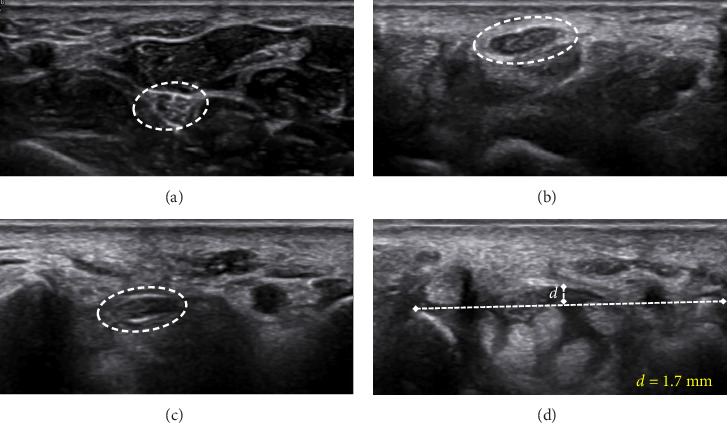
Transverse ultrasound image of the right median nerve in a female patient with mild CTS. (a) CSA at the pronator quadratus muscle (CSAp) is 0.06 cm^2^. (b) At the carpal tunnel inlet, CSA increased to 0.11 cm^2^. (c) The CSA at the carpal tunnel outlet is 0.09 cm^2^. (d) Bowing of the flexor retinaculum at the inlet is 1.7 mm.
